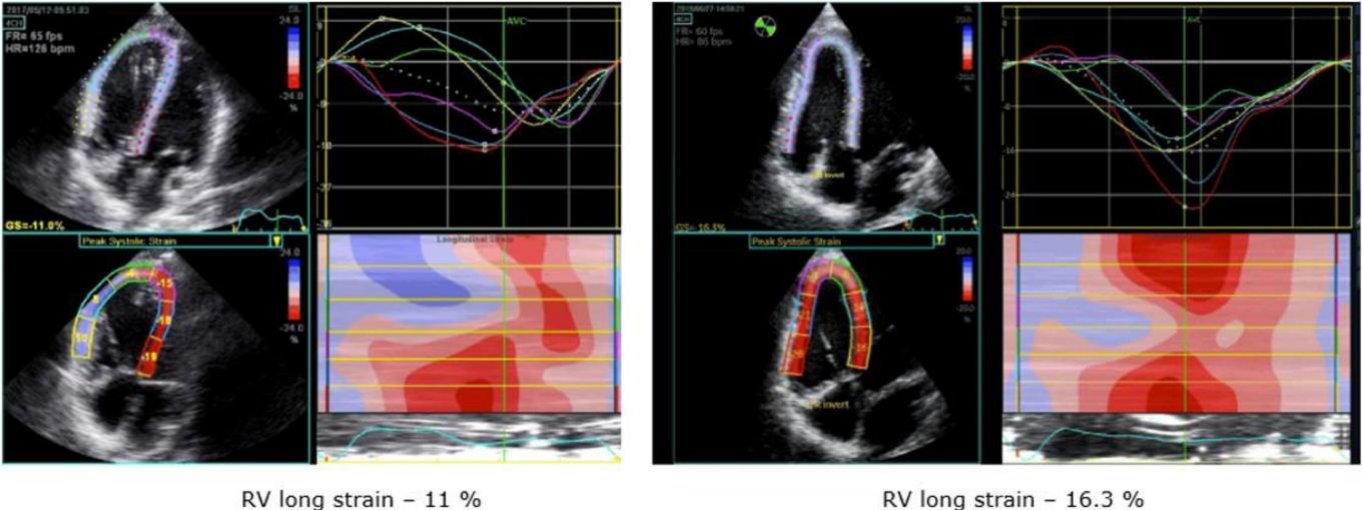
Stress echocardiogram performed at 50 W before and 4 months after pulmonary valve replacement in footballer.
